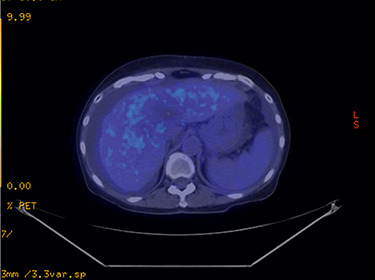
Axial PET CT demonstrating complete metabolic response post brachytherapy.
